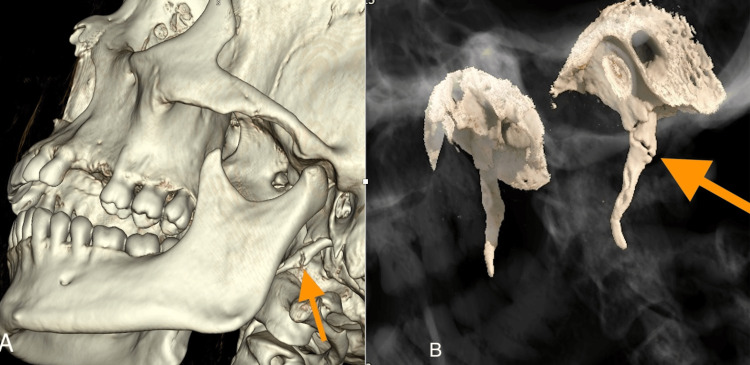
3D reformatted CT images. (A) and (B) Detailed visualization of the fracture surfaces is provided with 3D images (yellow arrows). CT: computed tomography.
